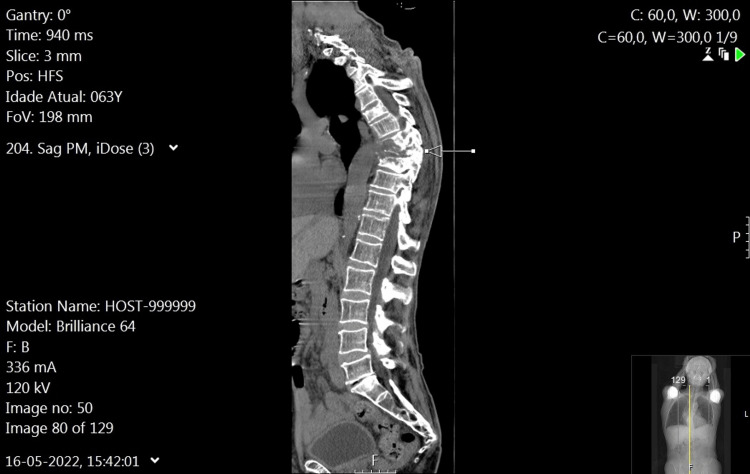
Spinal CT scan (sagittal section) In the sagittal section, a lytic lesion is observed centered at T8, with subtotal destruction of the bodies of T7 and T8, and the upper part of the body of T9. This is associated with erosion of the left pedicle of T8 and the ipsilateral costovertebral joint. There is a marked component with soft-tissue density in the perivertebral region between T6 and T9, with intracanal extension and molding of the adjacent spinal cord surface, and apparent foraminal extension to the right between T6-T7 and T9-T10, and to the left between T7-T8 and T8-T9.
